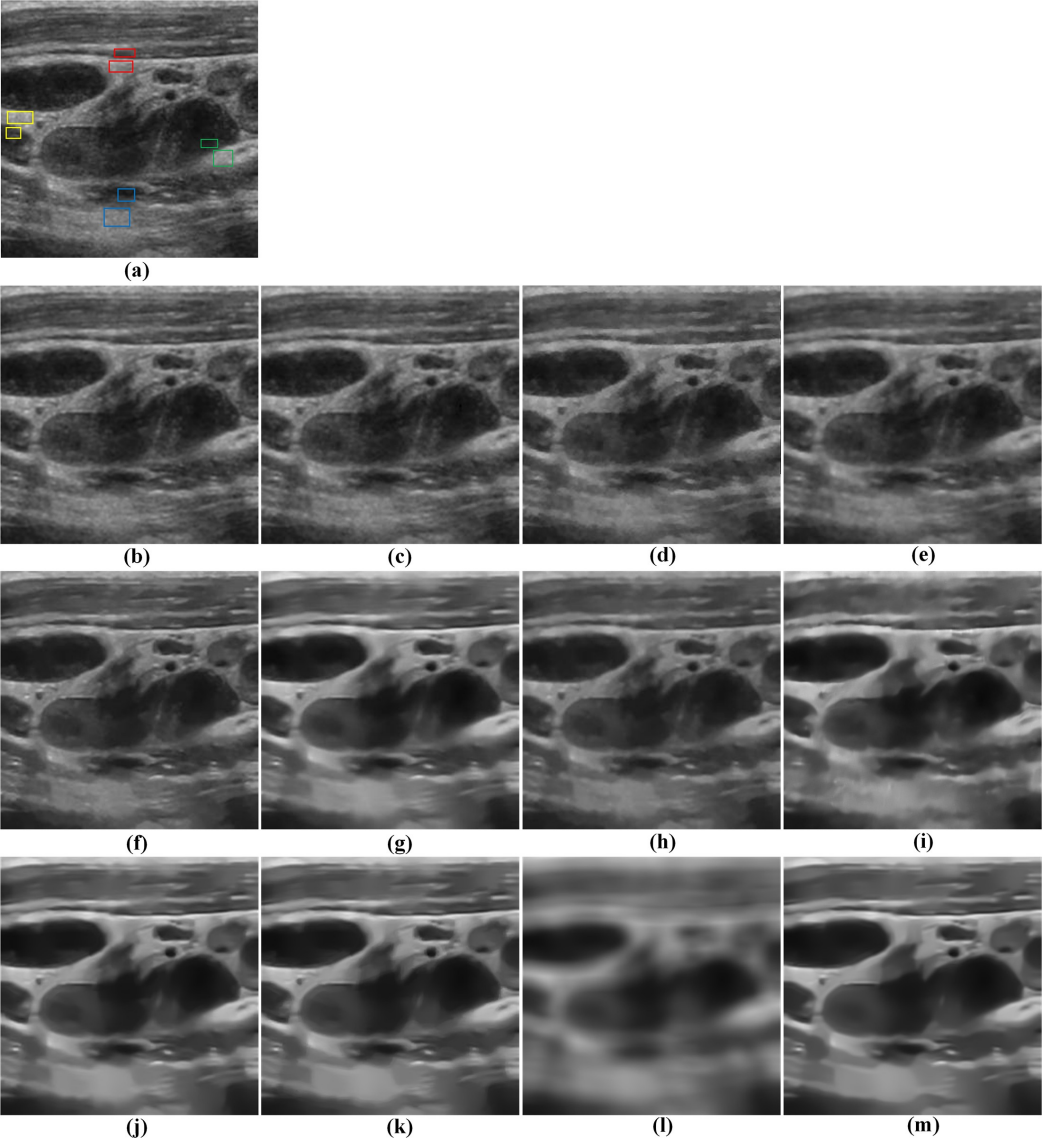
Comparison of restoration performance of various despeckling methods on a real ultrasound image of benign lymph nodes. (a) original noisy image and four ROIs marked with the different colors, (b) Frost filter, (c) Kuan filter, (d) SBF filter, (e) SRAD filter, (f) TNLM filter, (g) OBNLM filter, (h) NLMLS filter, (i) DnCNN filter, (j) OPCA-NLM filter, (k) RPCA-NLM filter, (l) SPCA-NLM filter, and (m) PPCA-NLM filter.
